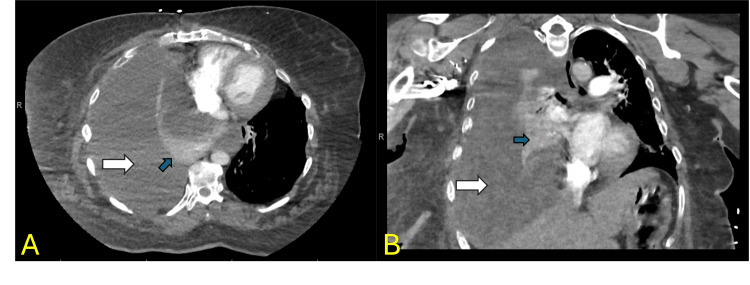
CT scan of the chest Axial section (A) and coronal section (B) show large right pleural effusion (white arrows) with complete atelectasis of the right lung (blue arrows) CT: computed tomography
